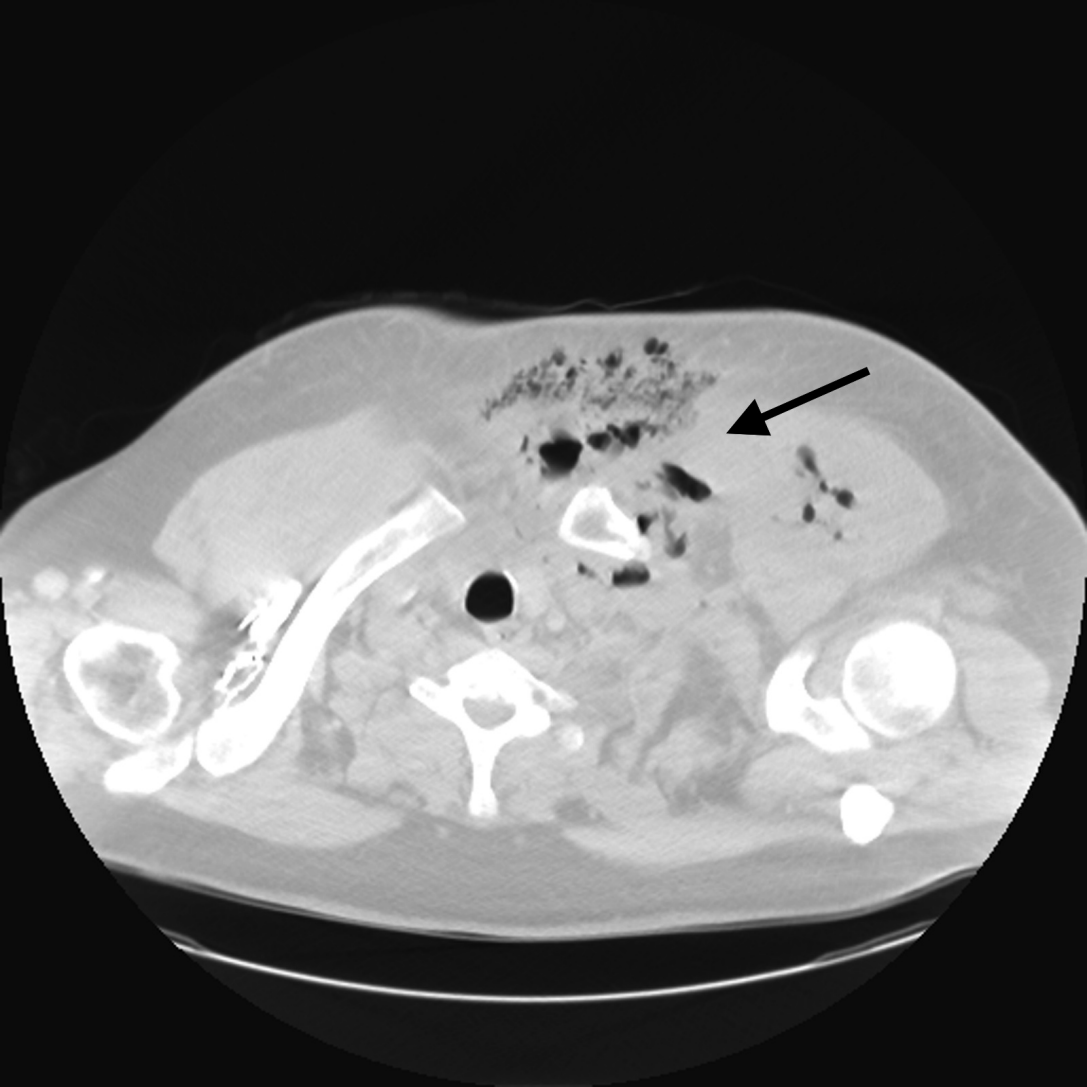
Chest computed tomography with intravenous contrast demonstrating chest wall collection extending into the anterior mediastinum
